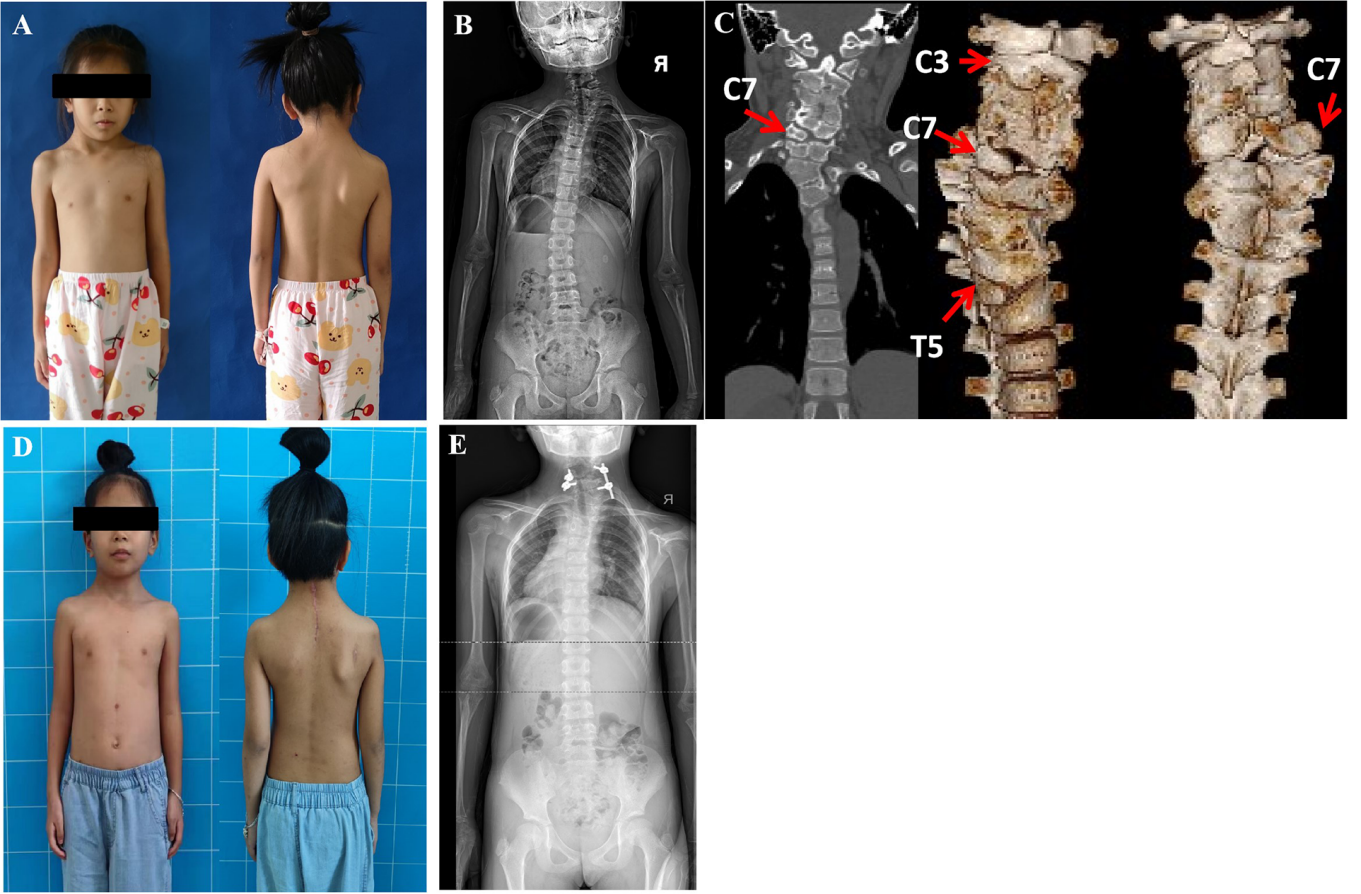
An 8 years 4 months old girl could not gaze horizontally with rigid torticollis (A). 3D CT showed a complexed deformitu of Klippel-Feil syndrome: C3.C7.T5-HV-R,C7 segmented HV,C3,T5 semi-segmented HV; C4-C6-BV; synostosis: C2-C3-R; T4-T6-L (C). She underwent C7 HVR with bilateral short fusion and could gaze horizontally three months postoperatively (D). Radiographs images demonstrated that there was a congenital cervicothoracic scoliosis with a compensatory lumbar curve preoperatively (B), thereafter, the neck tilt was significantly improved and the compensatory lumbar curve became 0° straight postoperatively at the latest follow-up 4 months later (E).
